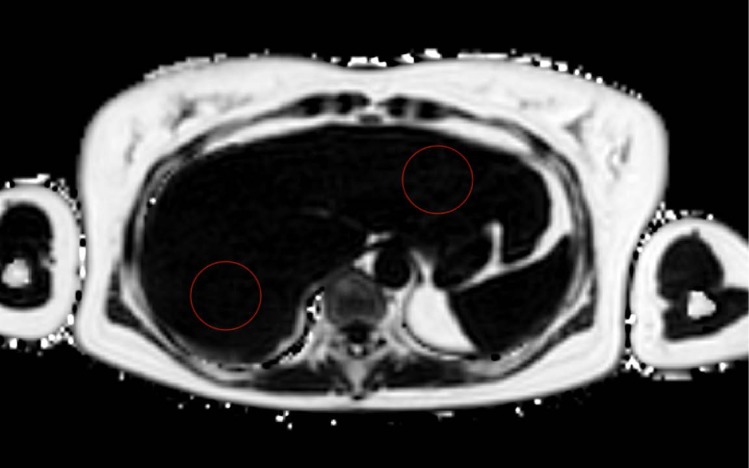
Example for the evaluation of the fat fraction using CSI-MRI (sequence: mDixon_Quandt, red circle: location of measurement)
